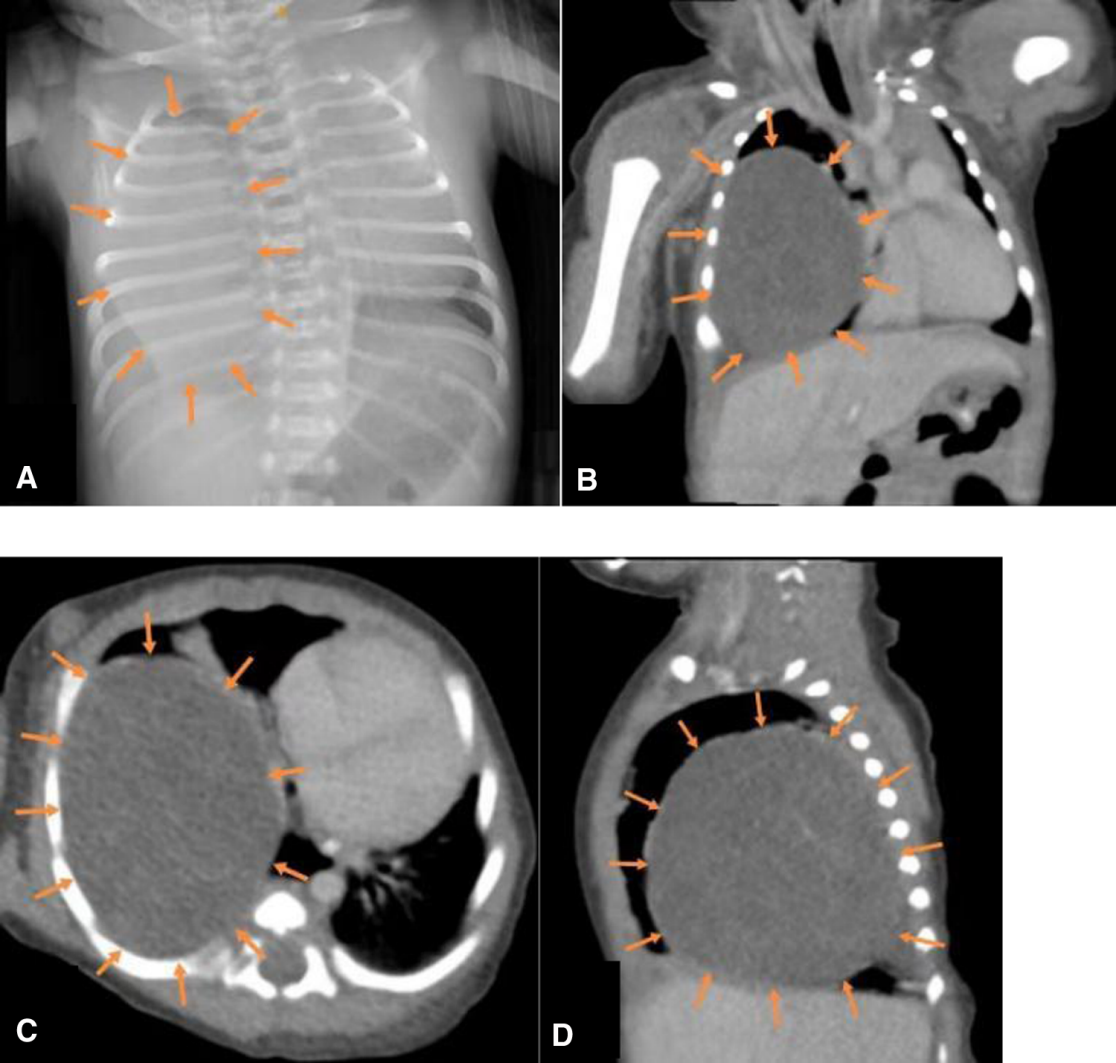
(A) x-ray and (B–D) CT (coronal, transverse and sagittal sections) showing a large mass in the right lower lobe.
